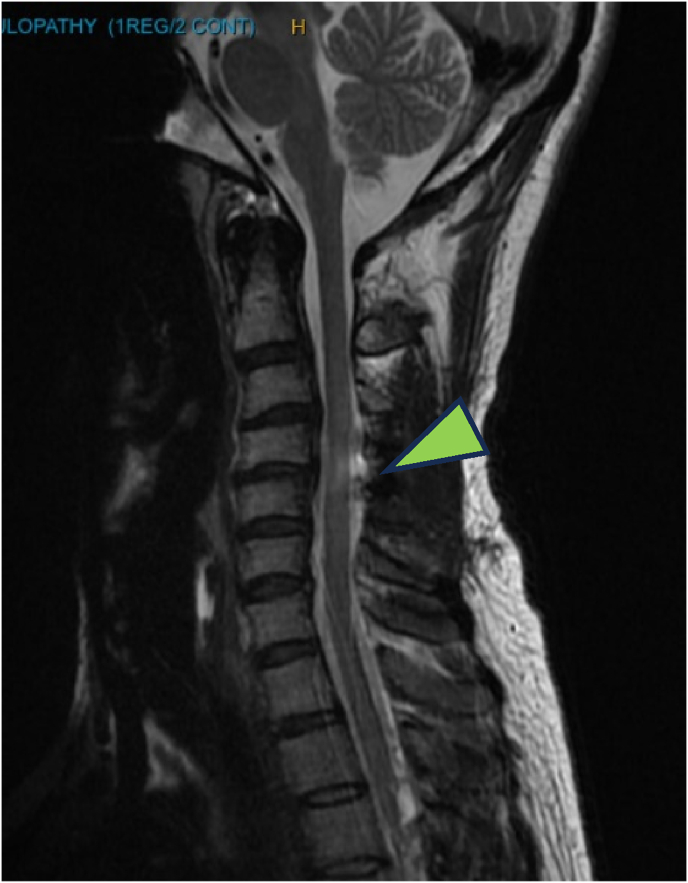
A 42-year-old male presented with over 10 yr history of neck pain and arm pain. MRI showed C4-5 disc herniation. (arrowhead).
